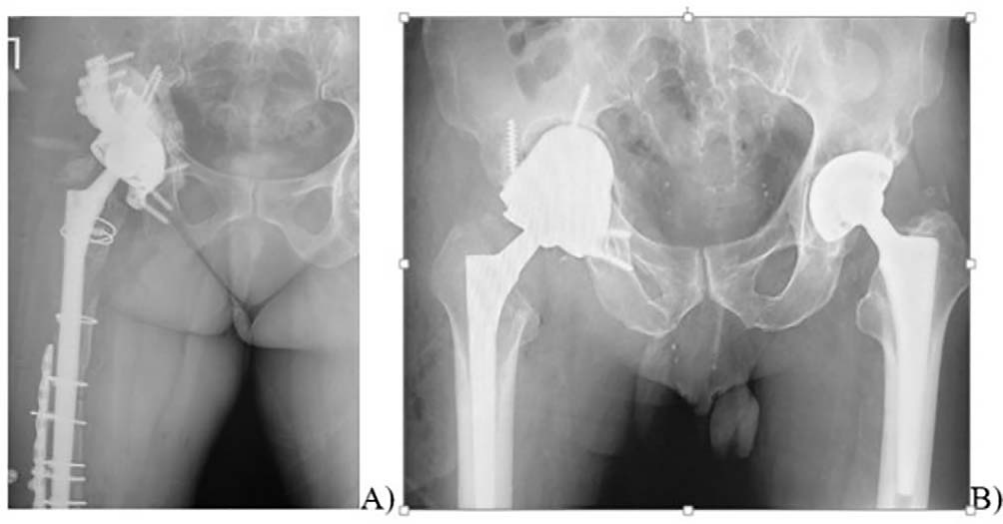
Radiographs of patients after revision hip arthroplasty: (A) 3D acetabular component with additional three-flange fixation. (B) 3D acetabular component without flanges (right).
